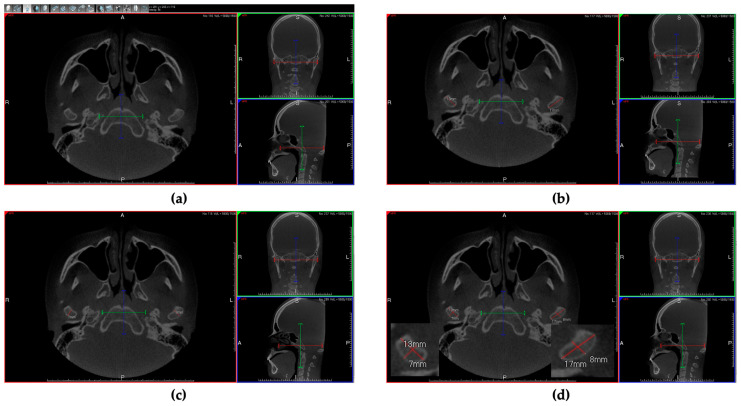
Example of the measurement’s protocols taken to assess the axial (AX_Diam) and transverse (TR_Diam) diameters: (a) slice of CBCT in which the condyle had its greatest transverse diameter in both coronal and axial projections; (b) considering the anterior and posterior poles of the condyle, the distance between these two points was measured by determining the length of the axial diameters of both condyles; (c) considering the medial and lateral poles of the condyle, the distance between these two points was measured by determining the length of the transversal diameters of the two condyles; (d) representation of both AX_Diam and TR_Diam of both right and left condyles.
